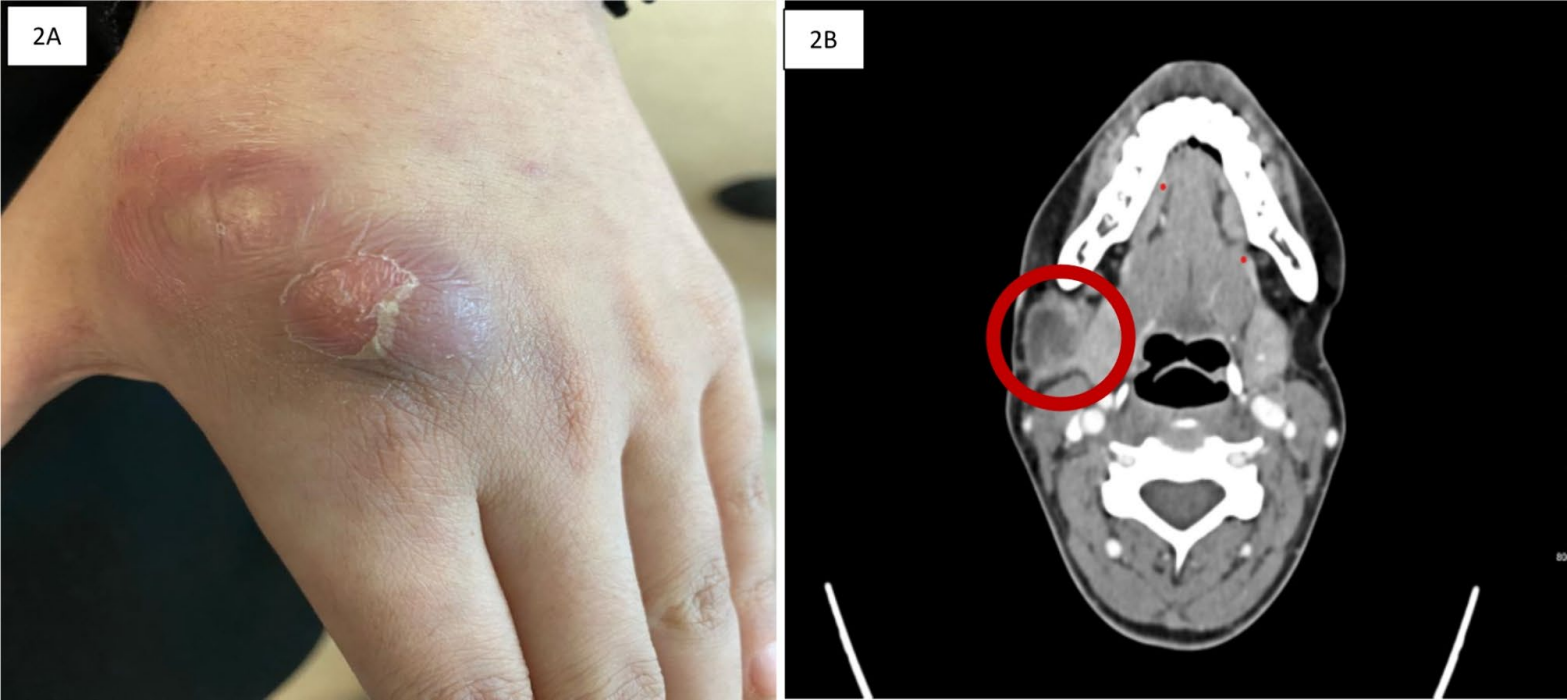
A: Appearance of a left-hand lesion after 4 weeks of restarting her on antituberculous treatment. Figure 2B: Enhanced CT scan of the neck four weeks after treatment with anti-TB agents showed an enlarged necrotic right submandibular lymph node
